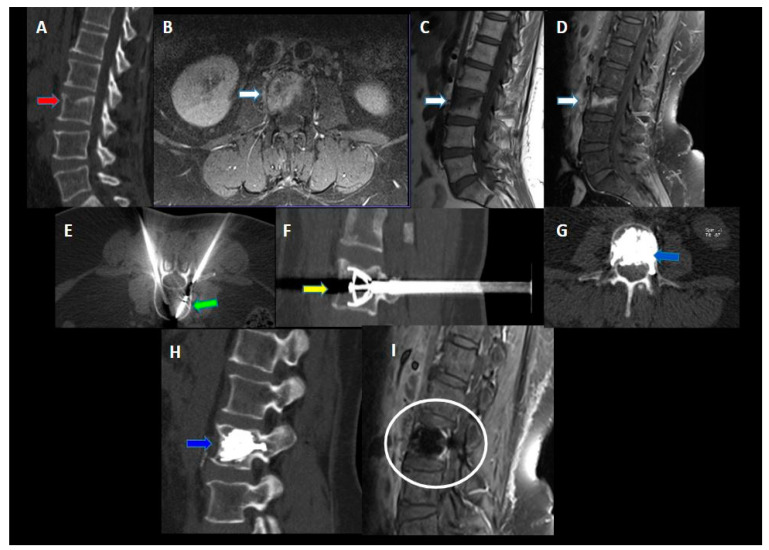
A 50-year-old female with a history of breast cancer with lumbar pain. (A) A CT of the lumbar spine in the sagittal plane shows a compression fracture of the superior endplate of the vertebral body of L3 (red arrow). (B–D) CE-MRI (in (B) the axial plane—CE T1 Fat-Sat sequence, in (C) the sagittal plane—T1 TSE sequence, and in (D) the sagittal plane—sagittal plane—CE T1 Fat-Sat sequence) confirms the presence of active vertebral fracture associated with a hyperintense focal lesion in T1 Fat-Sat sequences (white arrows). (E) Axial CT image; right microwave antenna inserted coaxially through the cannula for ablation (green arrow). (F) Sagittal MIP (maximum intensity projection) image after the opening of the SJ implants (yellow arrow). (G,H) Post-procedure control—CT in the axial (G) and sagittal (H) planes showed a correct expansion of the vertebra and a homogeneous cement filling (blue arrows). No procedural complications were observed. (I) follow-up MRI 12 months after the procedure (the sagittal plane-T1 Fat-Sat sequence): no evidence of local recurrence of the disease or complications in the treated vertebra (white circle).
